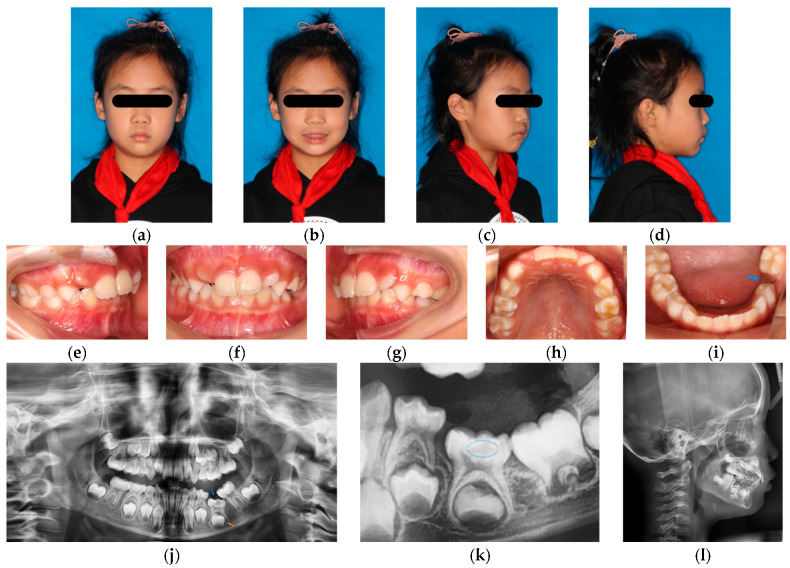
Initial facial photographs, intraoral photographs, and radiographic records of the patient. (a–d) Initial facial photographs. (e–i) Initial intraoral photographs showing mixed dentition with dental crowding and tilting of several teeth; the site of tooth 75 is indicated by the blue arrow in figure (i). (j) Initial panoramic radiograph showing severe infraocclusion of tooth 75 (blue arrow) with an indistinct periodontal ligament space at the mesial root and an intracoronal radiolucency extending into the middle layer of dentin beneath the enamel–dentin junction, and a close relationship between the root bifurcation of tooth 75 and the cortical bone of the 35 tooth bud (orange arrow); tooth 36 exhibits mesial inclination. (k) Radiographs obtained two years ago showing a radiolucency beneath the enamel–dentin junction of tooth 75 (blue dashed oval). (l) Initial lateral cephalometric radiograph.
